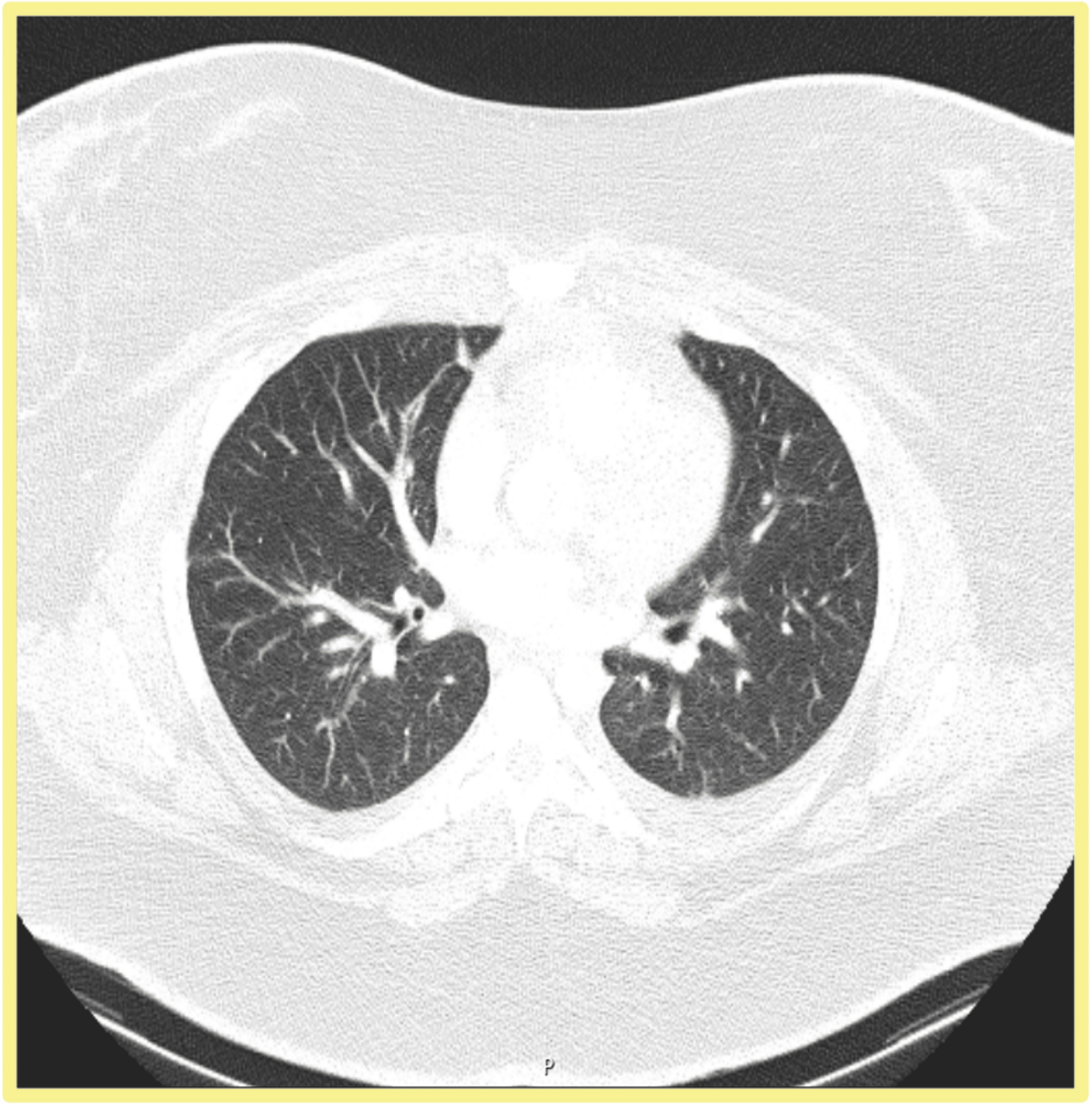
Axial CT of the thorax - lung window. The image demonstrates normal branching of the pulmonary vessels, with no evidence of pulmonary embolism or significant parenchymal abnormalities. No pleural effusion is observed in this slice.
